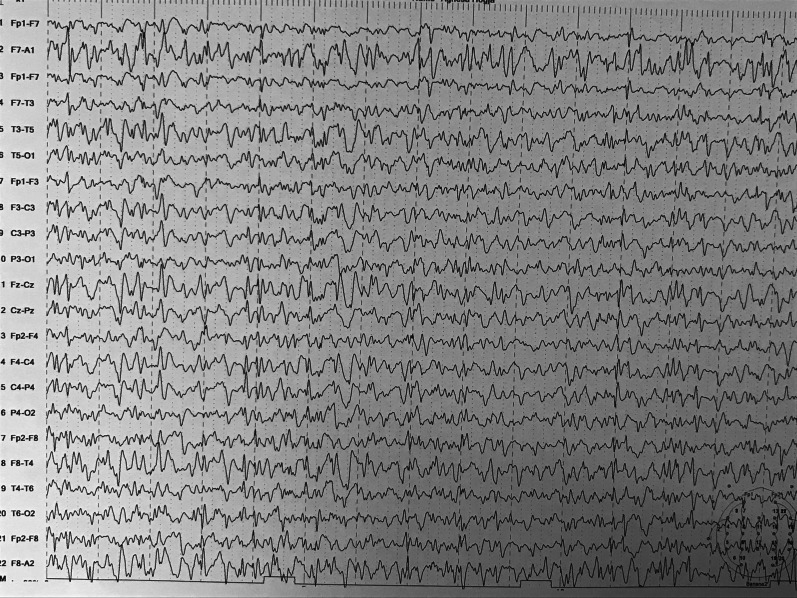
Follow-up Electroencephalogram (EEG) showing severe epileptic paroxysm activity in both hemispheres. Next, brain magnetic resonance imaging was planned (Fig. 3)
